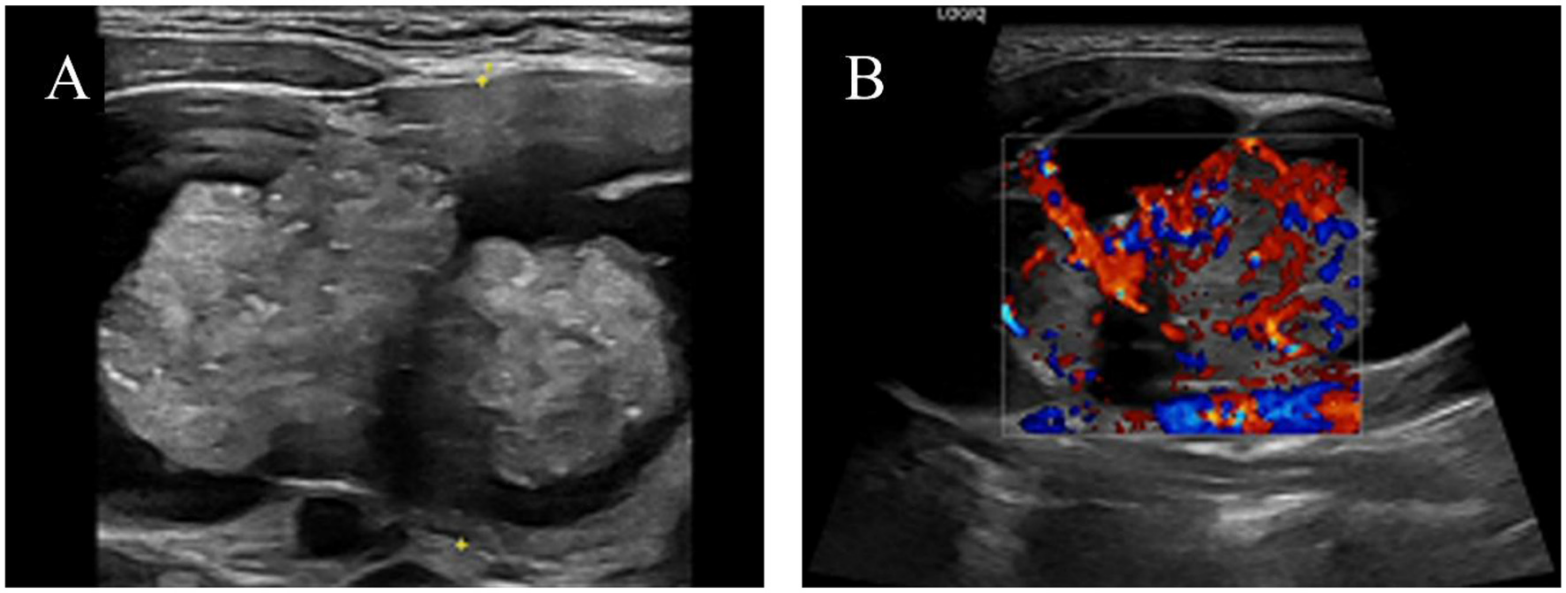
Color ultrasound images of the left thyroid lobe mass: (A) Cystic-solid mass; (B) Color Doppler flow imaging (CDFI) demonstrating abundant blood flow in the parenchymal portion.
